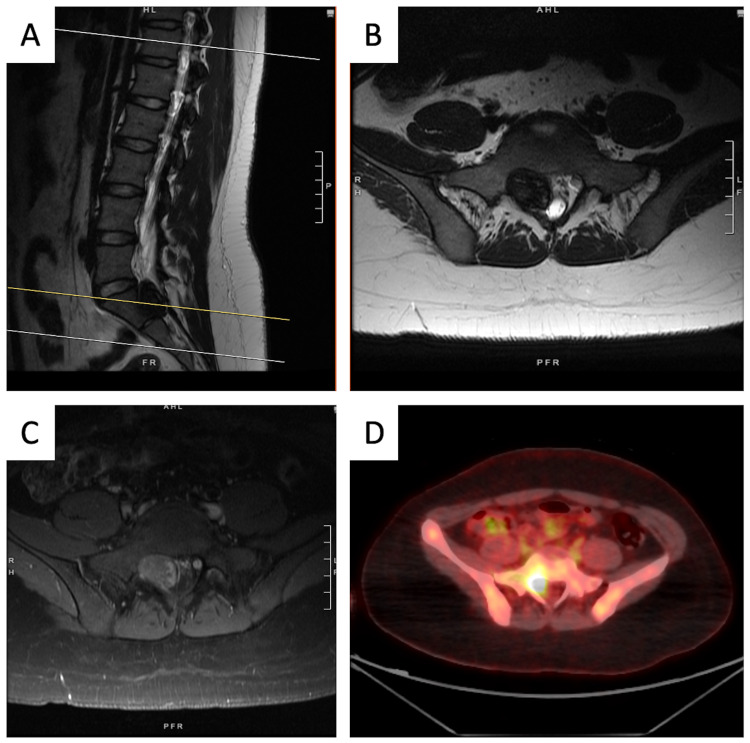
Preoperative imaging of right S1 malignant melanotic schwannoma. (A) T2 MRI sagittal showing T2 hypointense well-circumscribed lesion at the level of S1; yellow horizontal line demonstrates cross-cut for image (B) T2 MRI axial. The tumor appears primarily extradural and extends into the neuroforamen. The lesion is also (C) T1 hyperintense on MRI and (D) highly avid on positron emission tomography/computed tomography (PET/CT).
